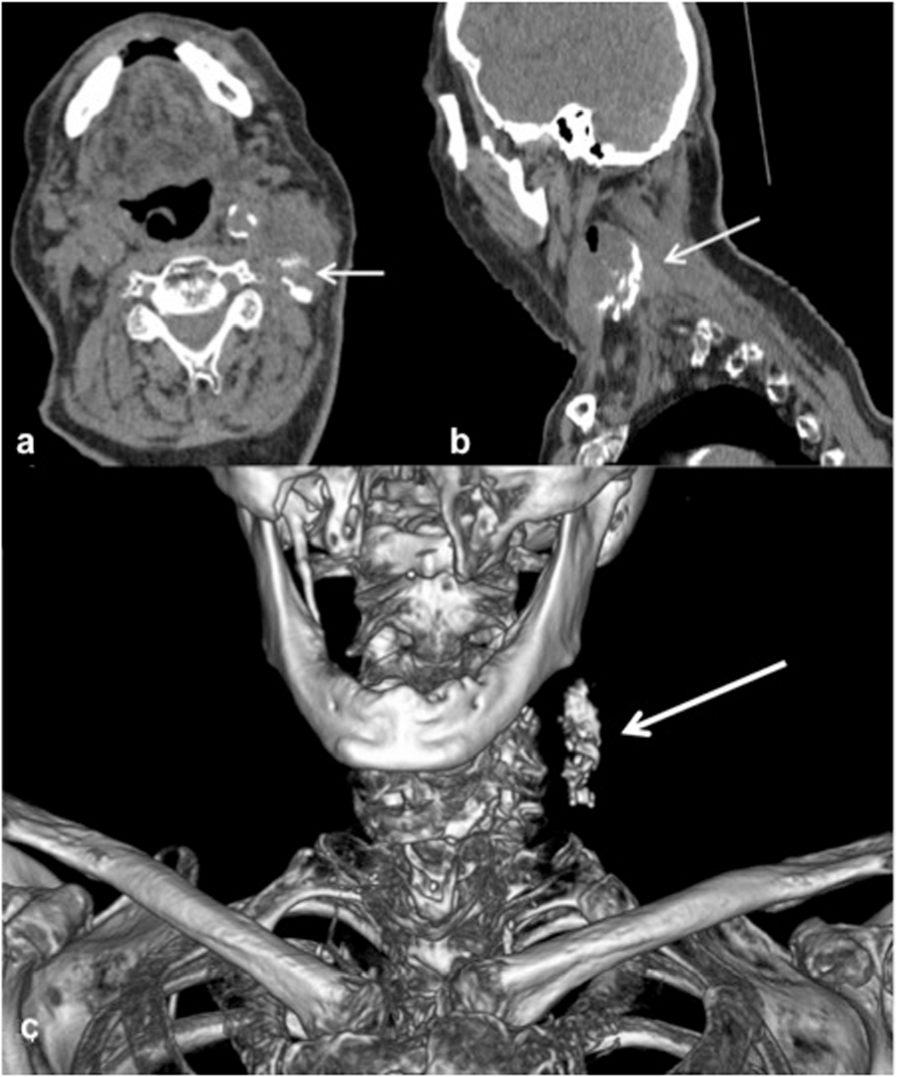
Computed tomography (CT) images of patient number 1. Axial, sagittal, and three-dimensional CT reconstructions of patient number 1 with a large necrotic tumour on the left neck side. During injection, the holmium microspheres were clearly visible on ultrasound as a cloud in the necrotic fluid and did precipitate after some minutes at the bottom of the tumour. The dorsal and caudal accumulation of ^166^Ho microspheres in the tumour is also well visible in the three images obtained in supine position (arrows in a, b, and c)
